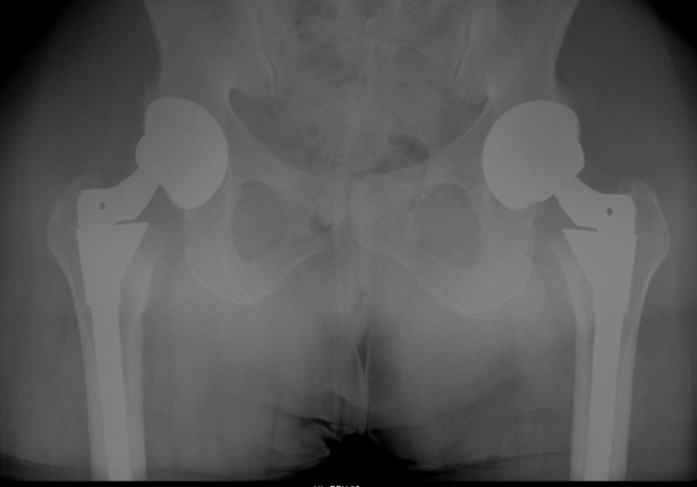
Preoperative radiograph obtained at the time of patient’s initial presentation. All arthroplasty components are in acceptable position without evidence of wear or loosening.
